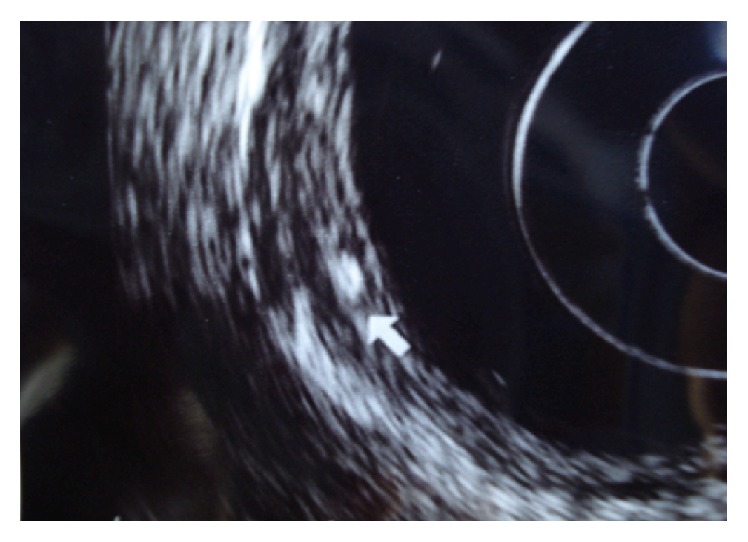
Endoscopic ultrasound image of a submucosal granuloma with calcified egg in its interior (white arrow).
